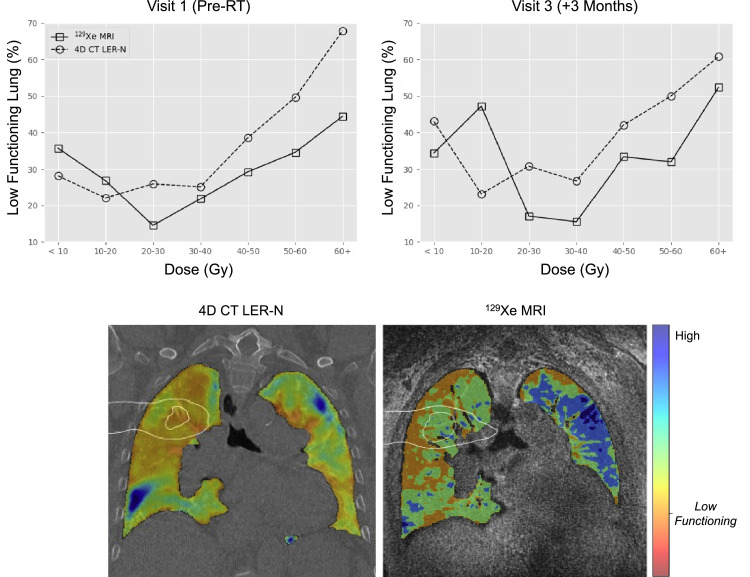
4DCT and HP-Xe MRI-derived ventilation comparison. Ventilation metrics derived by 4DCT LER-N using 10 different respiratory phases and HP-Xe MRI were compared prior to RT and 3 months following RT. Results were reported as the percentage of low-functioning lung volume within each 10-Gy isodose region (top). Ventilation maps 3 months after therapy comparing functional lung derived by 4DCT (bottom left) and HP-Xe MRI (bottom right) are shown, with blue indicating high-functioning lung and orange indicating low-functioning lung; 20-Gy and 10-Gy isodose curves are displayed (white outline overlay). *Abbreviations:* 4DCT = 4-dimensional computed tomography; LER-N = N-phase localized expansion ratio; MRI = magnetic resonance imaging; RT = radiation therapy.
